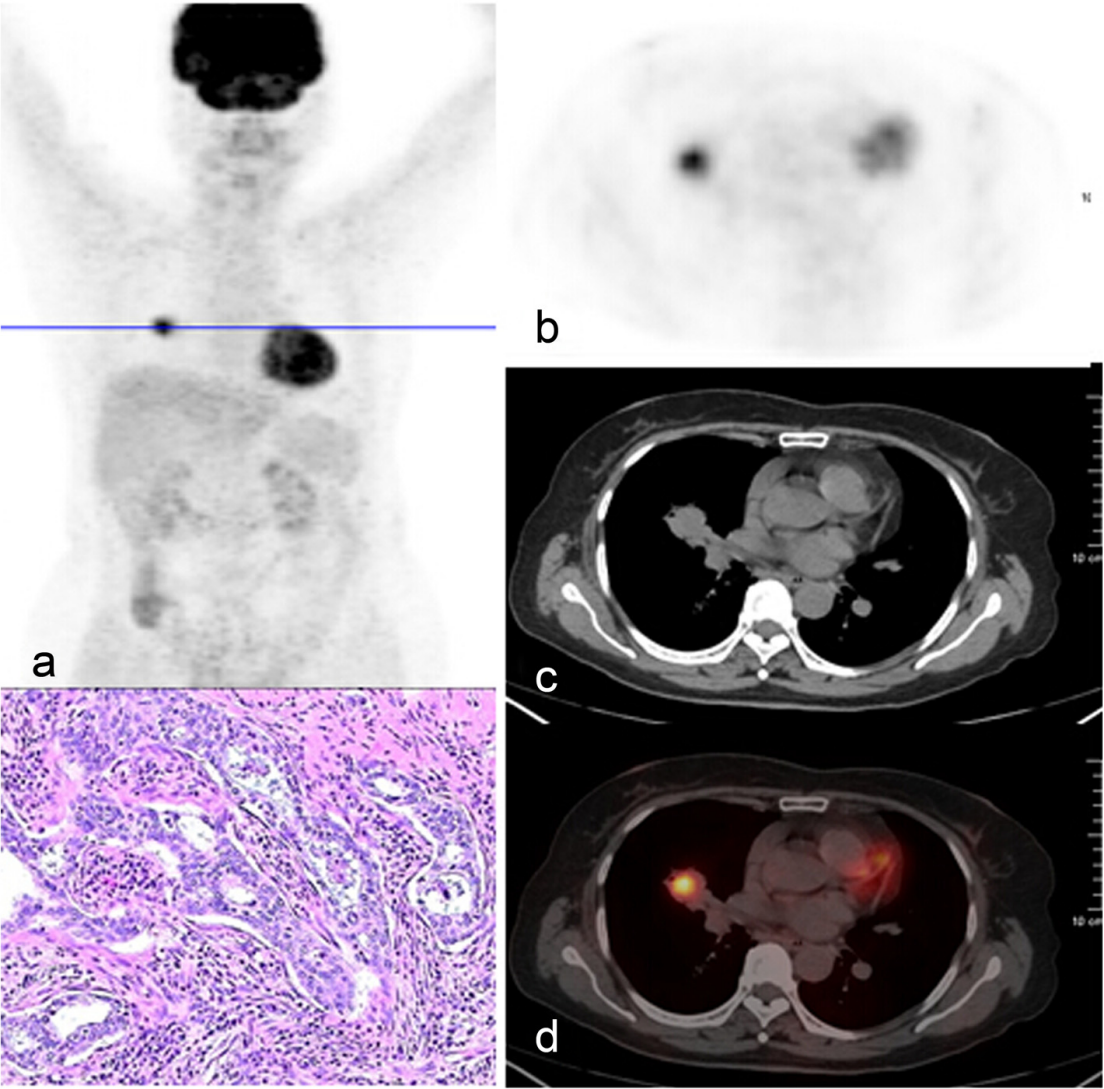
Full ^18^F-FDG uptake nodule in a 68-year-old female. The whole-body PET (a) and axial PET (b), CT (c), and fused PET/CT images (d) show FDG accumulation in the nodule of the middle lobe of the right lung. The pathologic result was adenosquamous carcinoma
